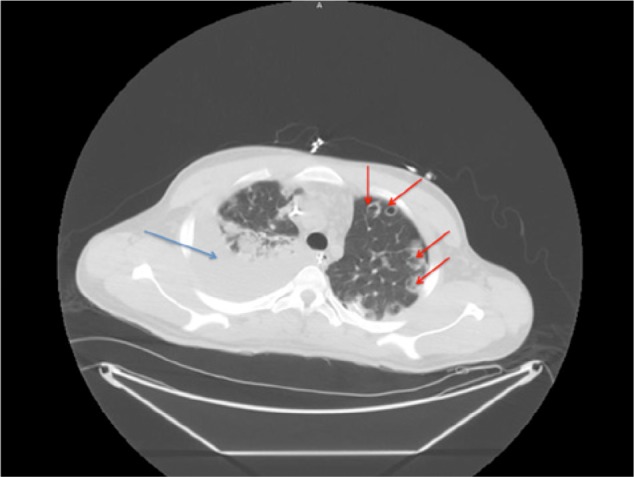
Computed tomography scan of the chest showing infiltrate (marked as blue arrow) and multiple small cavitatory lesions (marked as red arrow) in bilateral lung.
